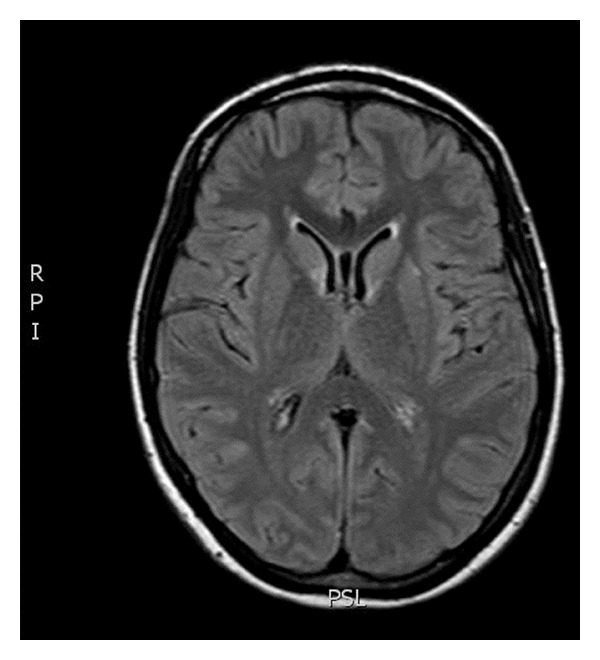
Brain MRI (T1-weighted image) was repeated and showed marked decrease in the volume of cerebral edema following 10 days of therapy with prednisone. Note the increased space of the gray-white matter junction and the ventricles (arrows).
